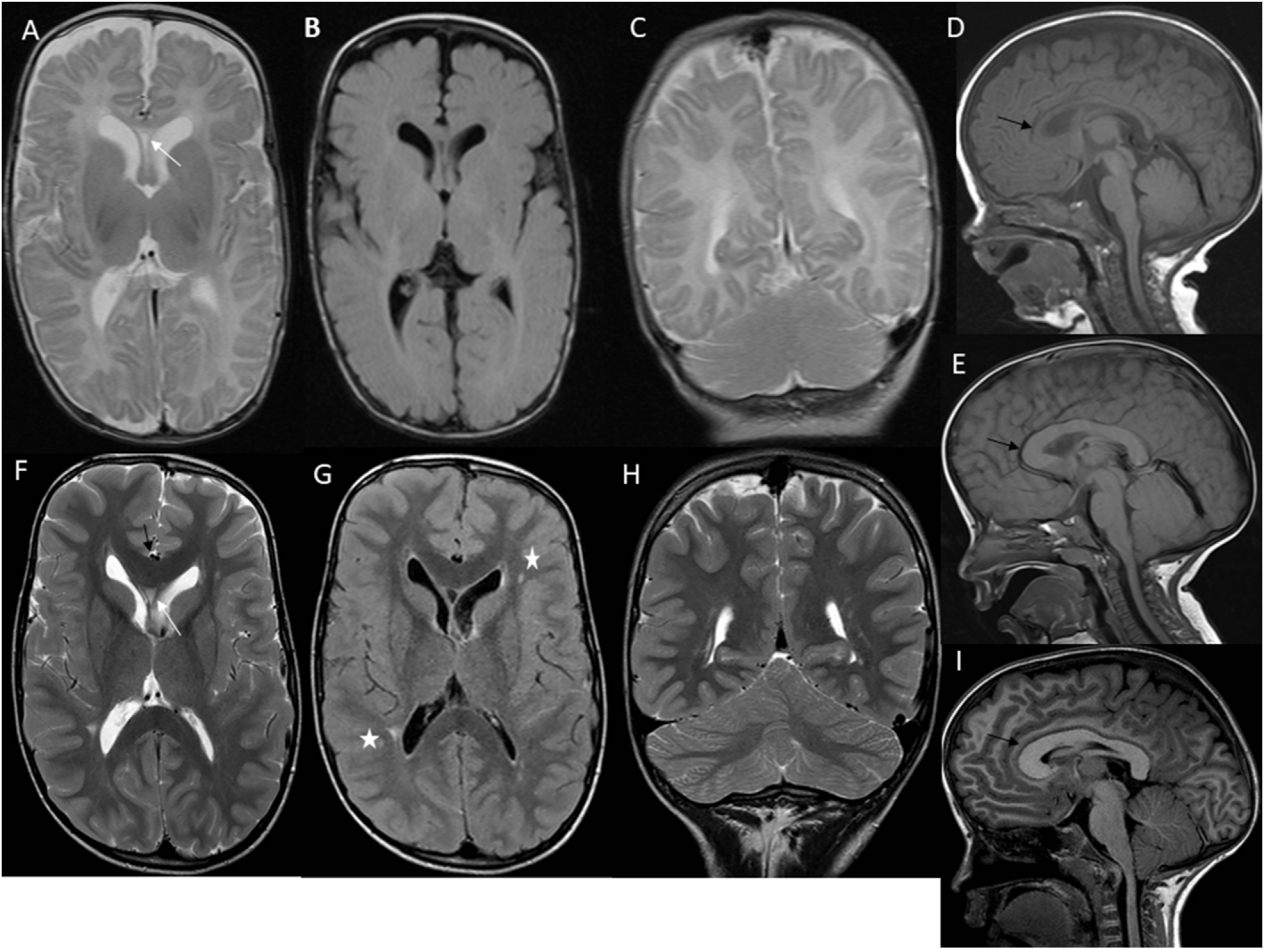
First case imaging series at different ages. At 2 months, imaging reveals megalencephaly, mild ventriculomegaly, and asymmetric development of cerebral and cerebellar hemispheres, along with a septum pellucidum cyst (white arrow) (A–C). Sagittal T1 W image (D) shows an extended frontooccipital distance (150 mm, >97th percentiles) and an elongated corpus callosum (black arrow) (anteroposterior diameter 63 mm, >97th percentiles) with normal thickness. At 3 years, there is a persistent elongation of the frontooccipital distance and a thick corpus callosum (>97th percentiles) (black arrow) (E). At 7 years, axial T2 W TSE image (F) shows ongoing megalencephaly, pronounced ventriculomegaly, asymmetric cerebral hemispheres, a small septum pellucidum cyst (white arrow), and a thick corpus callosum (black arrow) with normal myelination. Axial T2 W FLAIR image (G) highlights asymmetric ventriculomegaly and high signal focal changes in the periventricular white matter (white asterisks). Coronal T2 W TSE image (H) and sagittal T1 W image (I) reveal persistent asymmetry in cerebral and cerebellar hemispheres, extended frontooccipital distance (202 mm, >97th percentiles), mild cerebral tonsillar herniation (black arrow), and significant elongation and thickness of the corpus callosum (anteroposterior diameter 93 mm, >97th percentiles).
